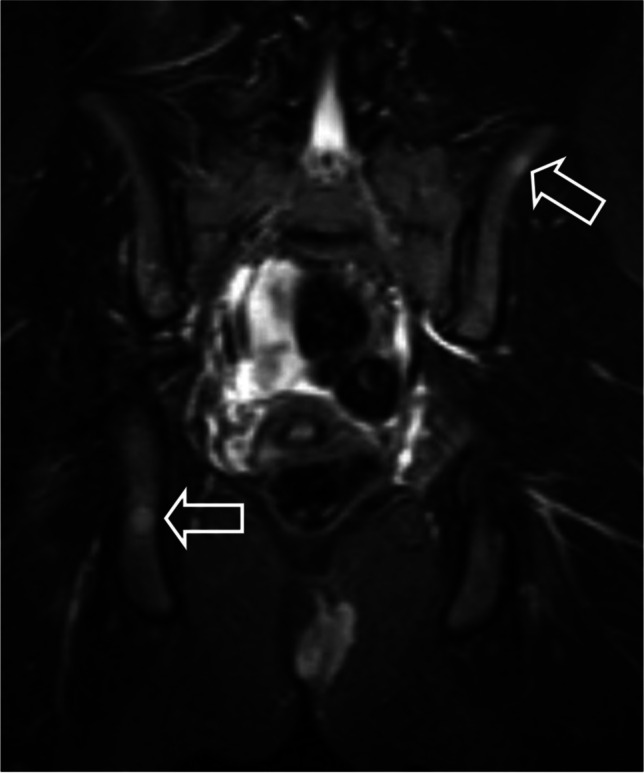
Coronal short tau inversion recovery magnetic resonance image of the pelvis in a 14-year-old girl (patient 2) shows diffuse osseous metastases (*arrows*)
